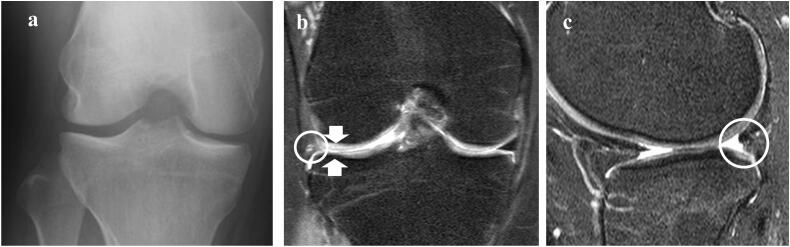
Preoperative images. a Rosenberg view X-ray of the lateral compartment shows a lateral joint space width of 2.9 mm. b Coronal magnetic resonance imaging (MRI) demonstrates the near complete disappearance of the middle part of the lateral meniscus (white circle) along with cartilage damage (white arrow). c Sagittal MRI shows that the posterior part of the lateral meniscus has almost disappeared (white circle).
